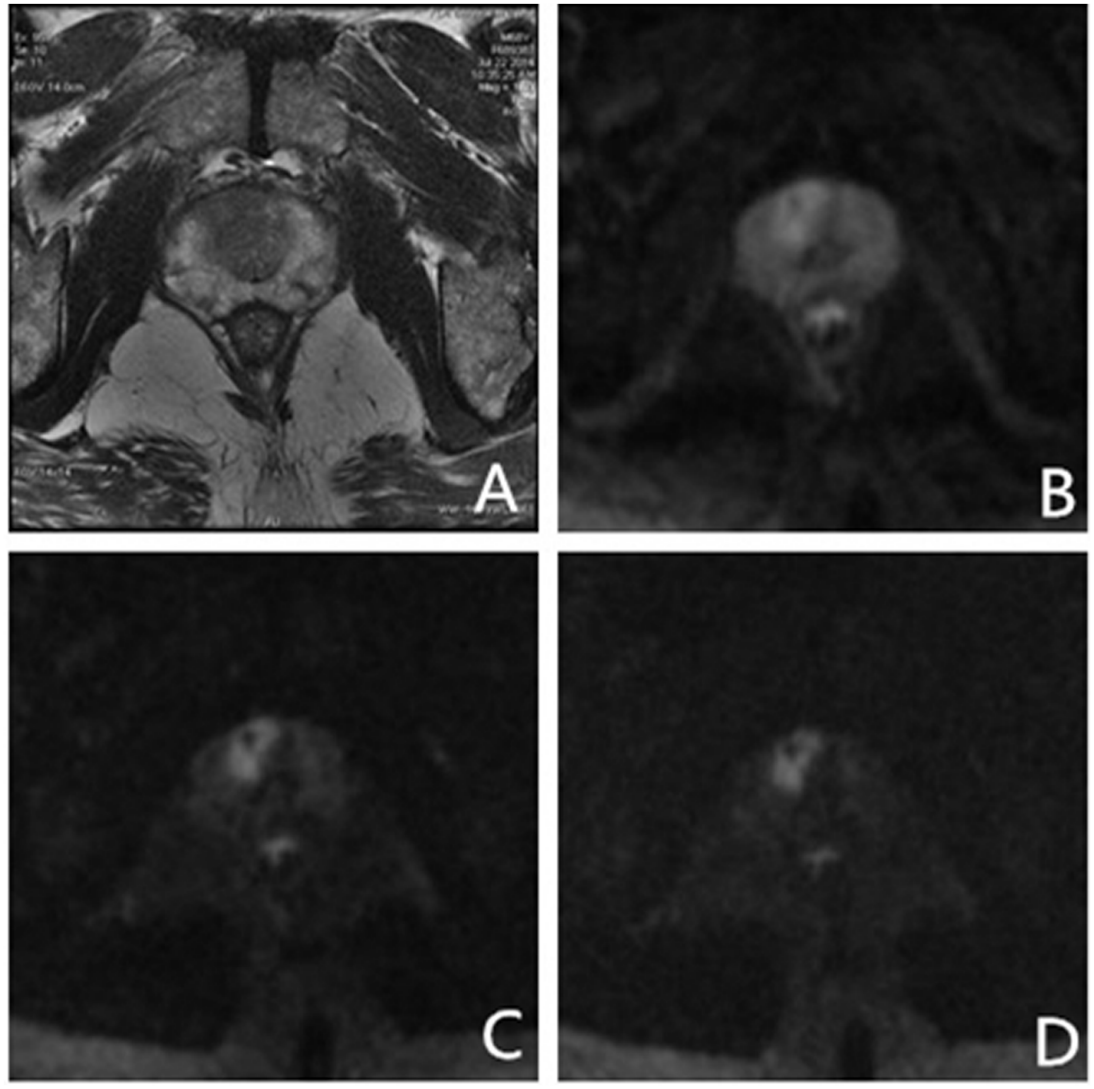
Images of a 68 yr old male patient with total PSA 10.5 ng/ml and free PSA 1.66 ng/ml. Systematic transrectal biopsy confirmed PCa (Gleason score 4 + 3 = 7). (A) High-solution T2-weighted imaging shows low signal intensity area in the right TZ, and PI-RADS score of 2 due to subtle mass effect; (B) PI-RADS score of 3 on DWI with b-value 1000 s/mm^2^. The cancer is ambiguous because of high signal intensity from surrounding parenchyma; (C, D) PI-RADS score of 5 on ultrahigh DWI with b-value 2000, 3000 s/mm^2^. High signal intensity areas in the right portion of the TZ are clearly visible. Key: PSA—Prostate specific antigen; PCa—prostate cancer; TZ—transition zone; DWI—diffusion-weighted imaging; PI-RADS—Prostate Imaging Reporting and Data System.
